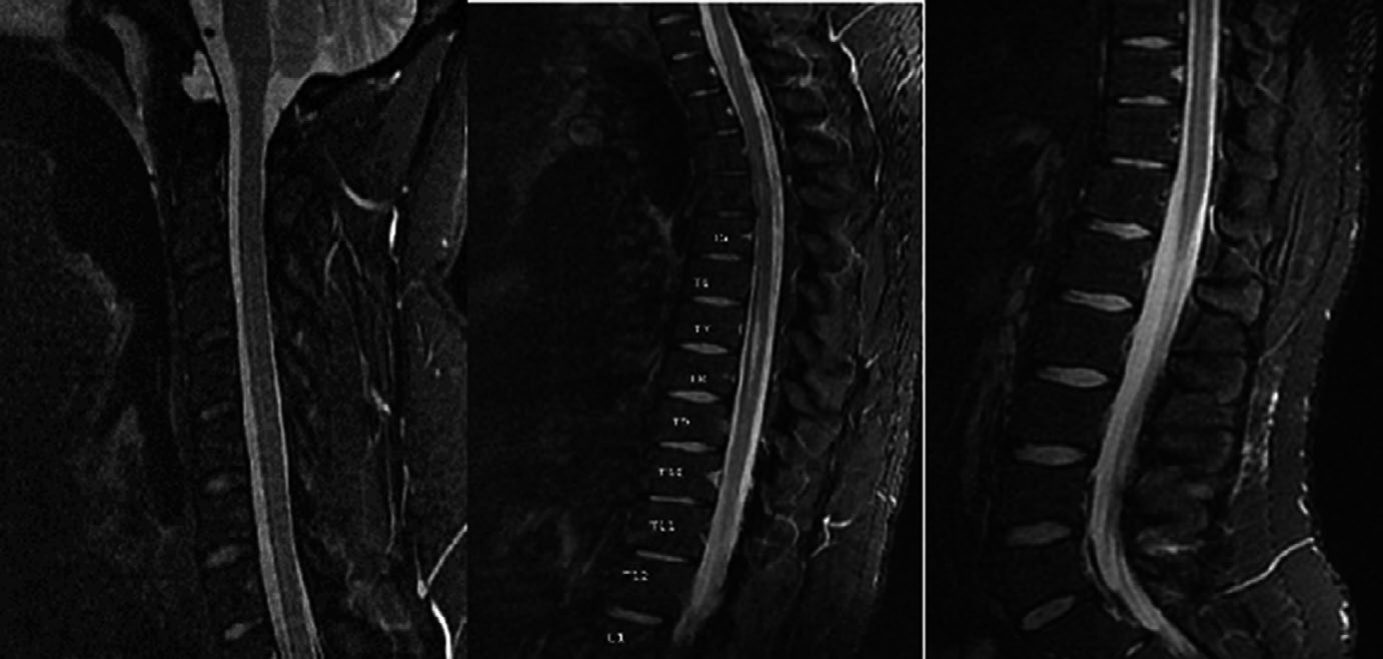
During admission, contrast MRI of cervicothoracic STIR sequences did not show any medullary pathology
